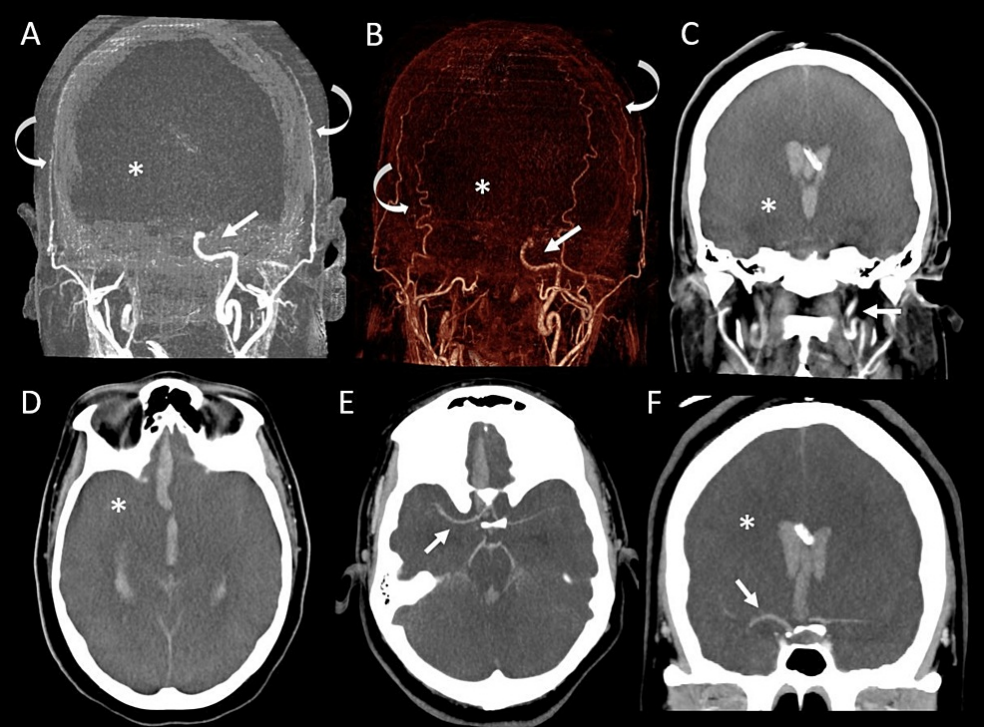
Arterial phase head computed tomography (CTA) Arterial phase CTA head in coronal subtracted (A) maximum intensity projection (MIP) and coronal volume rendered image (B) and coronal (C) and axial (D) MIP demonstrate lack of opacification of the intracranial arteries (asterisk in A, B, C, D) beyond the left cavernous internal carotid artery; although there is contrast opacification of the external carotid artery branches (curved arrows in A, B) likely due to high intracranial pressure. Delayed postcontrast CTA in axial (E) and coronal (F) MIP demonstrates only minimal filling of the proximal intracranial arteries (arrows in E, F), which are diffusely narrowed with a lack of contrast opacification in the distal intracranial arteries (asterisk in F) representing diffuse cerebral swelling and high intracranial pressures suggesting early brain death.
